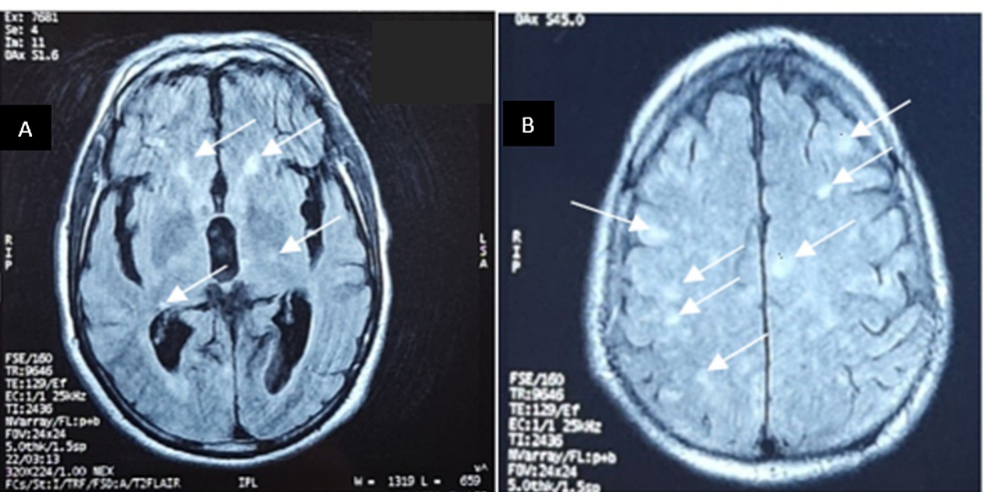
Diﬀuse hypersignal fluid-attenuated inversion recovery (FLAIR) lesions. (A) Periventricular areas. (B) Subcortical areas.
